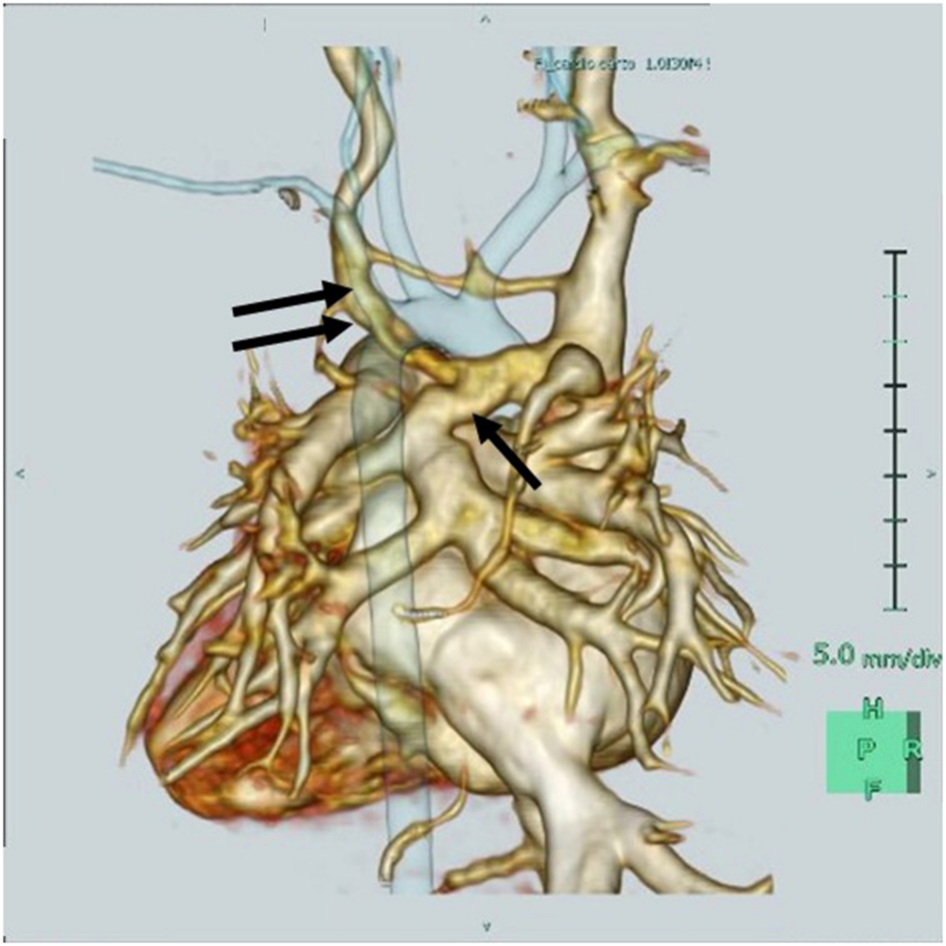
Three-dimensional cardiac computed tomography reveals that the retroaortic innominate vein (RAIV, double arrow) passes posterior to the ascending aorta to join the superior vena cava and the vertical vein (single arrow) merges into the RAIV.
